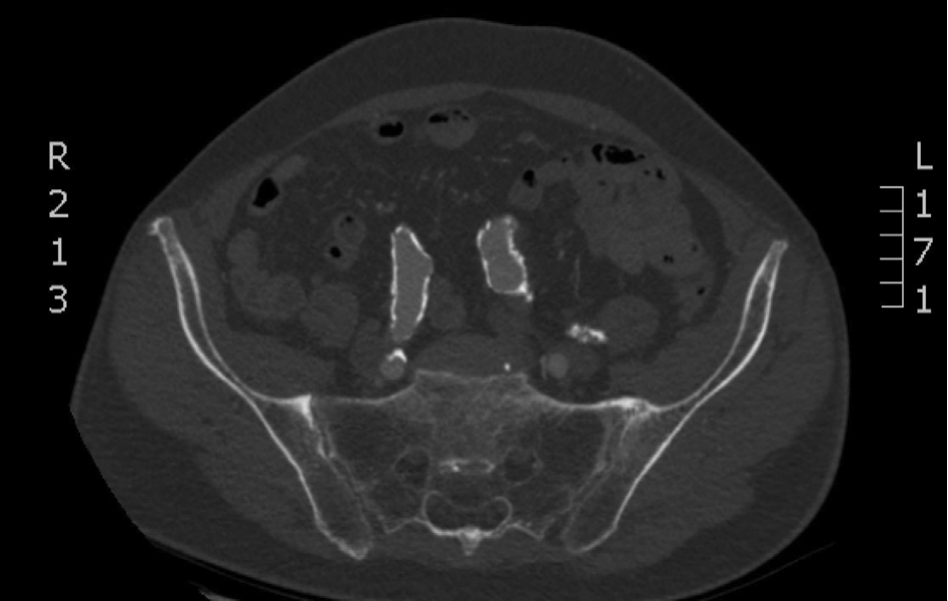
Axial computed tomography angiogram showing significant bilateral iliac artery calcification.
